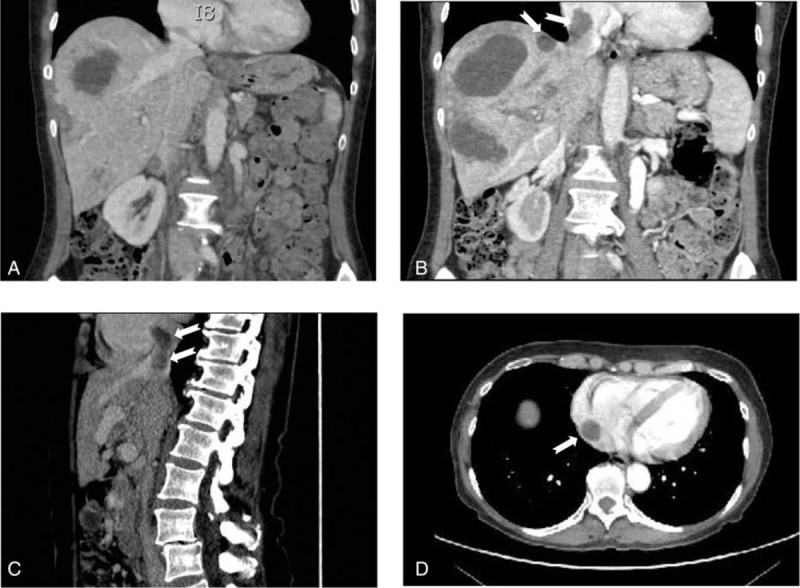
A thoracic and abdominal CT was performed before (A) and after 6 courses of bevacizumab (B, C, D). A (coronal reformatted CT): pretreatment imaging demonstrates multiple hypodense lesions in keeping with bilomas and permeable hepatic veins and inferior vena cava. B, C, D: following treatment, bilomas remain unchanged and thrombosis in the right hepatic vein (B, C), in the inferior vena cava (C) and in the right atrial cavity (D) were evidenced (arrows). CT = computed tomography.
